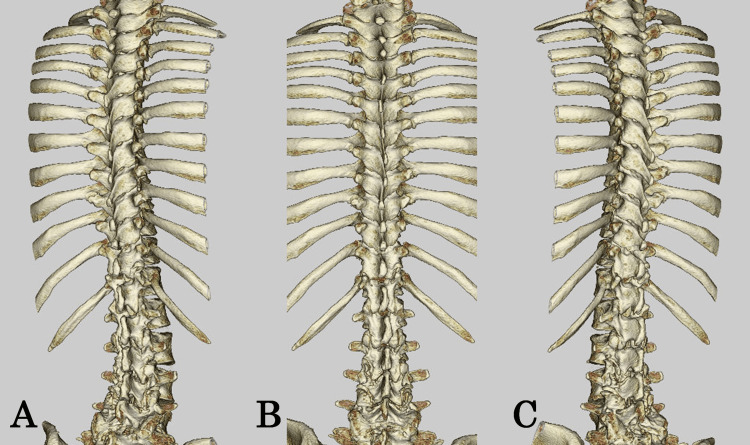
Thoracic spine CT (A) Right posterior oblique view, (B) posterior view, (C) and left posterior oblique view. No bony abnormalities or evidence of spinal dysraphism were observed. CT: computed tomography
